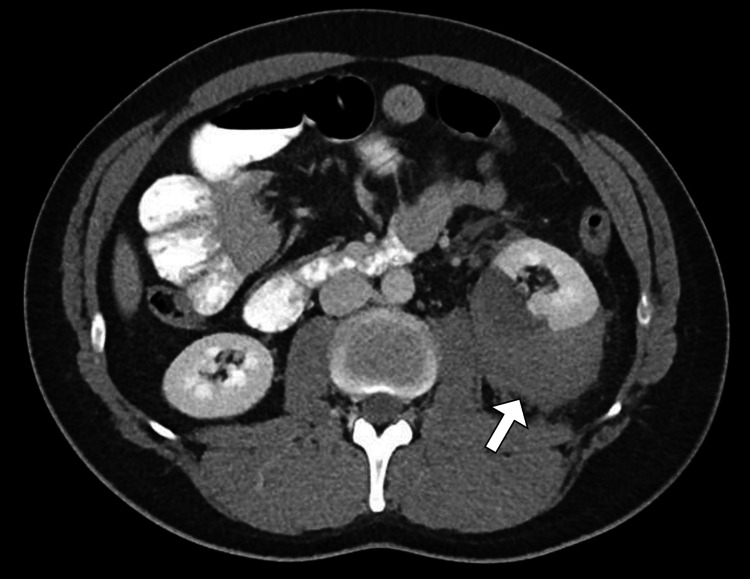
Left perirenal hematoma on axial CT. Axial contrast-enhanced CT image of the abdomen demonstrates a hyperdense left perirenal collection (arrow), consistent with a perirenal hematoma. This finding correlates with the ultrasound appearances and supports the diagnosis in the setting of idiopathic intracranial hypertension.
